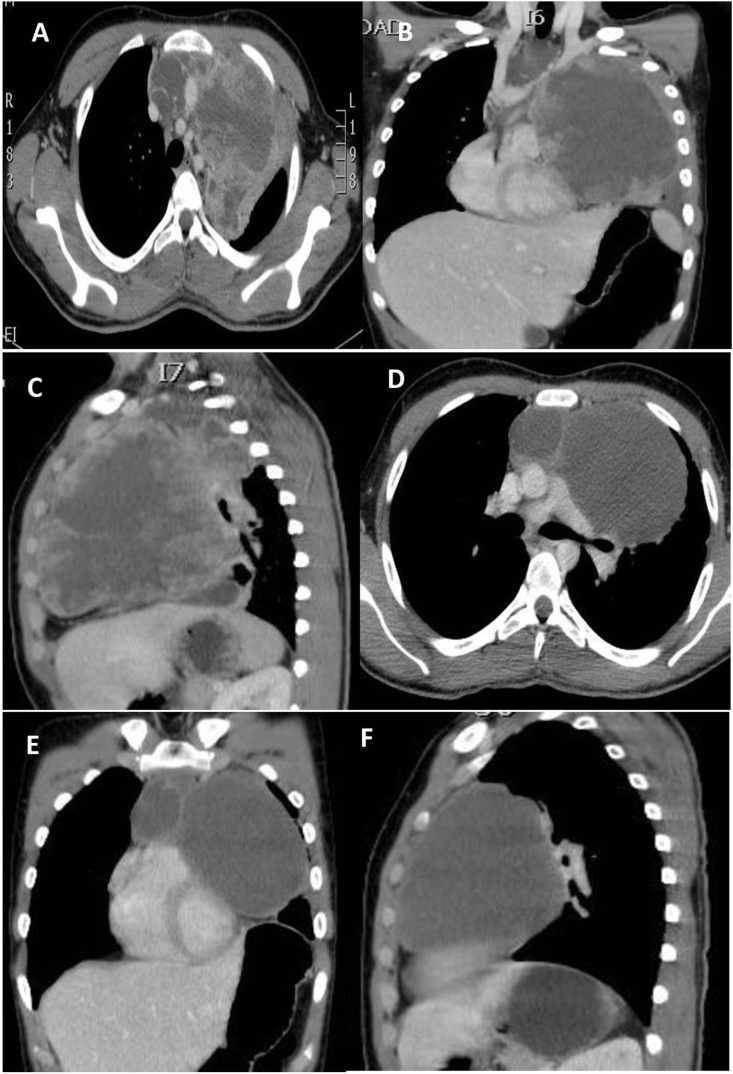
(A, B, C) CT scan of thorax Axial, coronal &Sagittal view showing large mediastinal mass mostly occupying the left hemi thorax. (D, E, &F) Post chemotherapy CT scan of thorax showing a large Residual anterior mediastinal residual mass.
